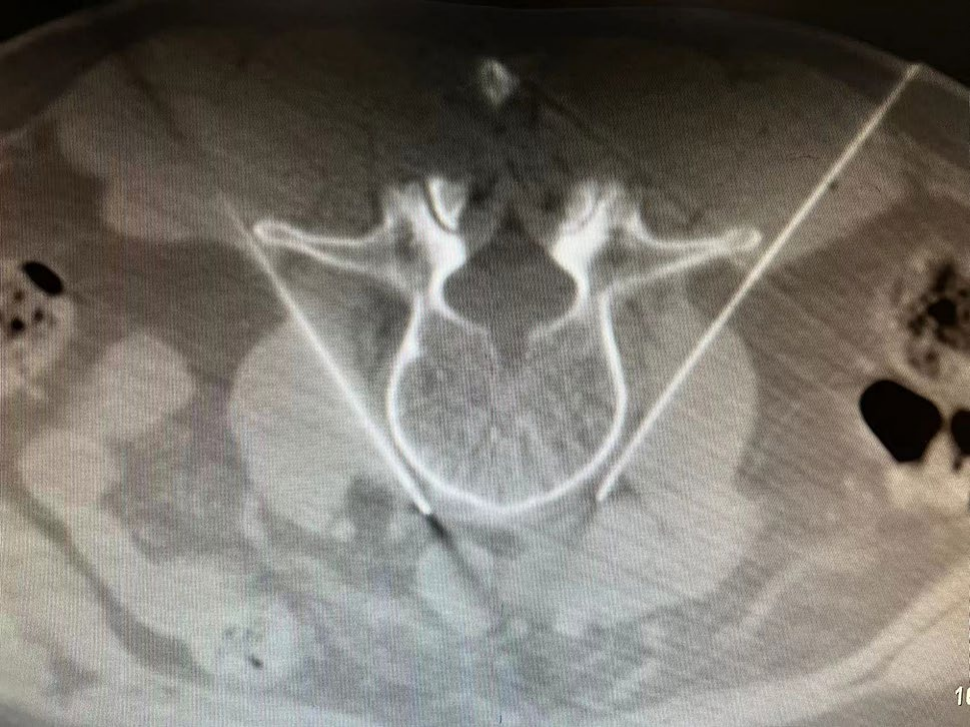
Radiofrequency thermocoagulation of bilateral lumbar sympathetic nerveStudies have shown [57–59] that CT-guided lower back sympathetic RF thermocoagulation has been gradually applied to the treatment of many autonomic nerve diseases, such as plantar hyperhidrosis (PH), cold hypersensitivity (CH), and diabetic peripheral neuropathy (DPN). Another study reported [60, 61] that lumbar sympathetic radiofrequency thermocoagulation achieved a lasting effect in the treatment of CRPS, with a pain relief rate of 91.7% at three months and 83.3% at six months after treatment. Radiofrequency thermocoagulation of the lumbar sympathetic nerve has a lasting and reliable effect on neuropathic pain in the lower extremities. It can improve blood supply to the lower extremities and relieve ischemia and pain symptoms to a certain extent.Unlike traditional sympathetic nerve block and chemical neuroregulation, radiofrequency thermocoagulation of the thoracic and lumbar sympathetic nervous systems locates the target nerve through electrical stimulation and uses high temperatures for thermocoagulation [62]. This process causes proteins to denature and coagulate, thereby blocking the transmission of nerve impulses. This method has the advantages of a precise destruction range, minimal damage, lasting efficacy, and high safety, and has been widely used by clinicians and patients.Dorsal root ganglion pulse radiofrequency combined with sympathetic radiofrequency ablationRadiofrequency ablation increases blood perfusion in the skin and muscles by reducing sympathetic nerve tension and increasing collateral and peripheral blood volumes. Pain stimulation cannot be transmitted through nerve fibers to the central nervous system, thereby inhibiting pain transmission and achieving the purpose of reducing neuropathic pain. PRF plays an analgesic role by regulating disturbed electrical signals around the diseased dorsal root ganglia and nerve fibers, downregulating substance P levels in the dorsal root ganglia, and upregulating substance P levels in the spinal cord. Sympathetic radiofrequency can effectively control intractable neuropathic pain, such as PHN, CRPS, and PRF, through different mechanisms of action. The combined application of these two methods for the treatment of intractable neuropathic pain warrants further exploration and research in clinical practice.
